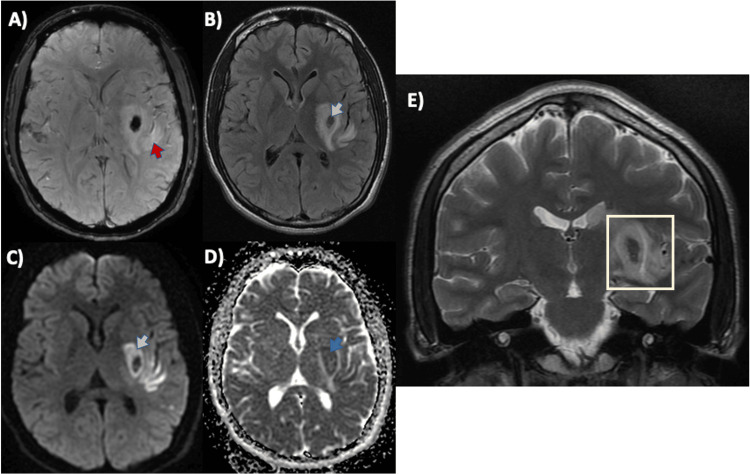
Brain MRI findings A) FLAIR sequence showing an area of peri-infarct edema (red arrow) and (B) magnetic susceptibility sequence demonstrating H2-type hemorrhagic transformation (ECASS), involving <30% of the infarct area (orange arrow). C, D) Diffusion (green arrow) and ADC (blue arrow) map showing diffusion restriction in the left lenticulostriate territory. E) T2 sequence delineating the affected left lenticulostriate region (yellow square) MRI: magnetic resonance imaging; FLAIR: fluid-attenuated inversion recovery; ADC: apparent diffusion coefficient
